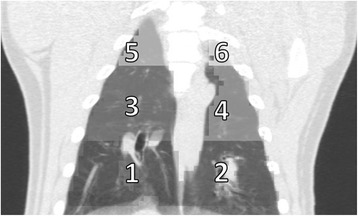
Coronal view of an in vivo CT image indicating the segmentation of the lungs performed for the regional validation against MS. Regions *1*–*2*, *3*–*4*, and *5*–*6* correspond to the basal, medial, and apical zones of the lungs, respectively
